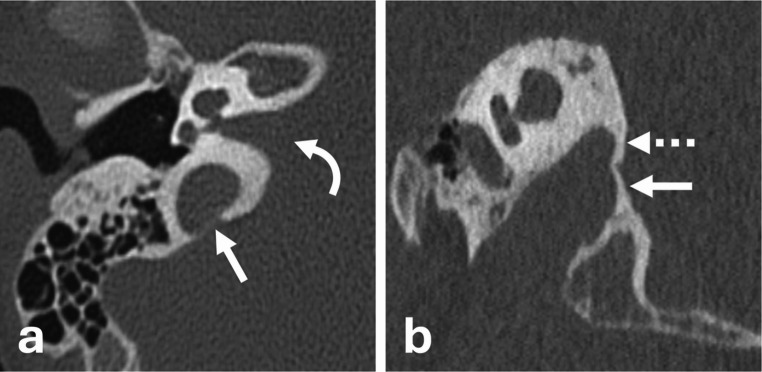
High-riding jugular bulb with diverticulum. (a) Axial CT showing a high riding jugular bulb (straight arrow) at the level of internal acoustic canal (curved arrow). (b) Sagittal CT showing a diverticulum (dashed arrow) at the tip of the high riding jugular bulb (solid arrow)
